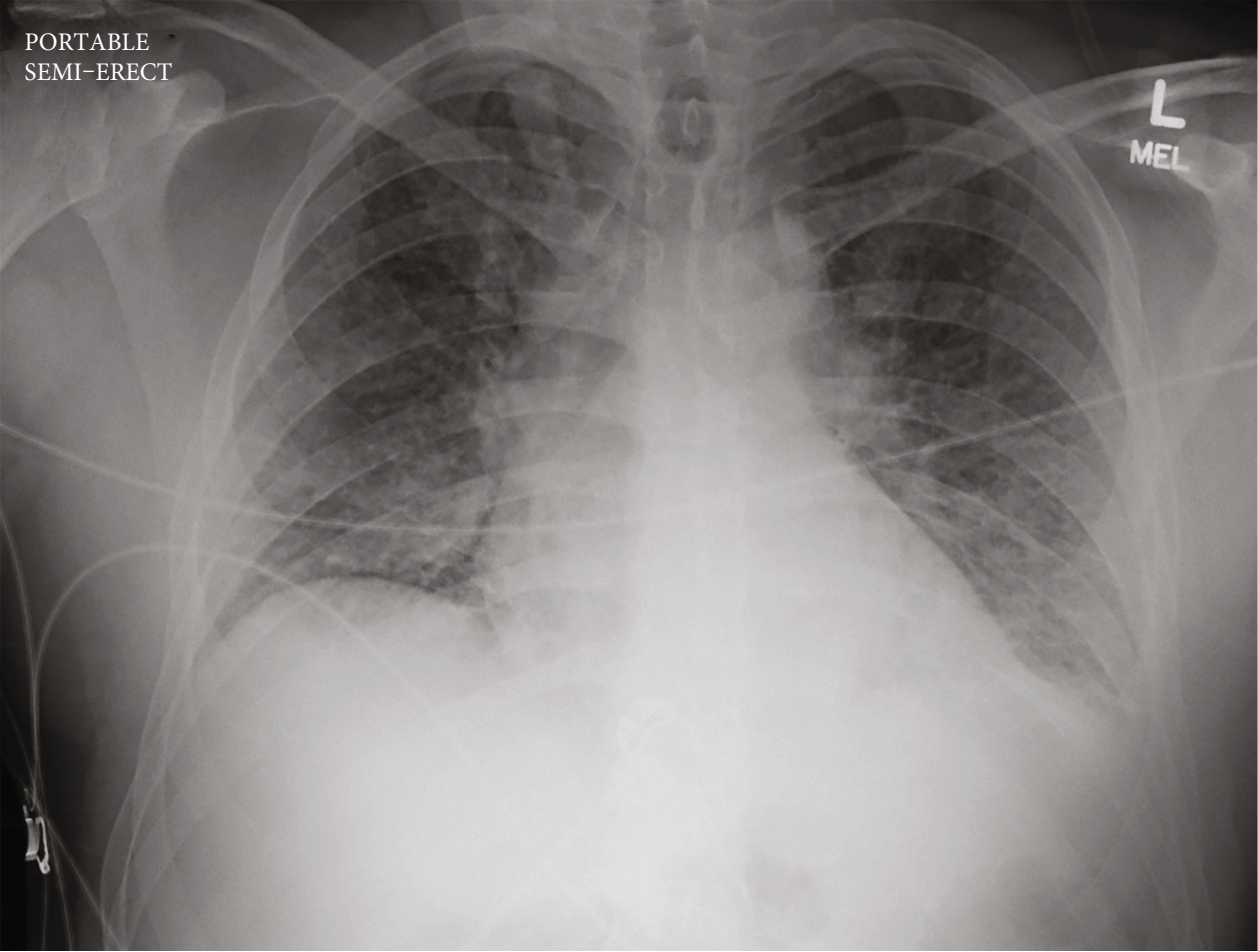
Chest X-ray on admission shows bilateral pulmonary infiltrates concerning for pulmonary edema vs. pneumonia.
